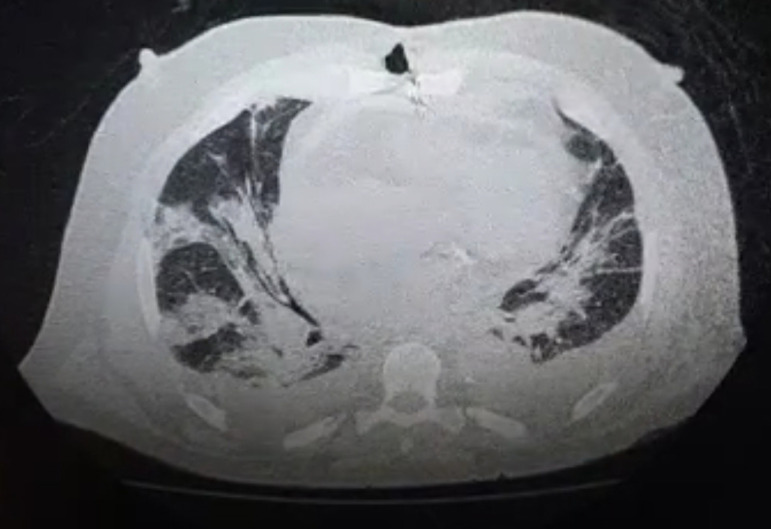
Chest CT revealing multiple peripheral ground-glass opacities.
